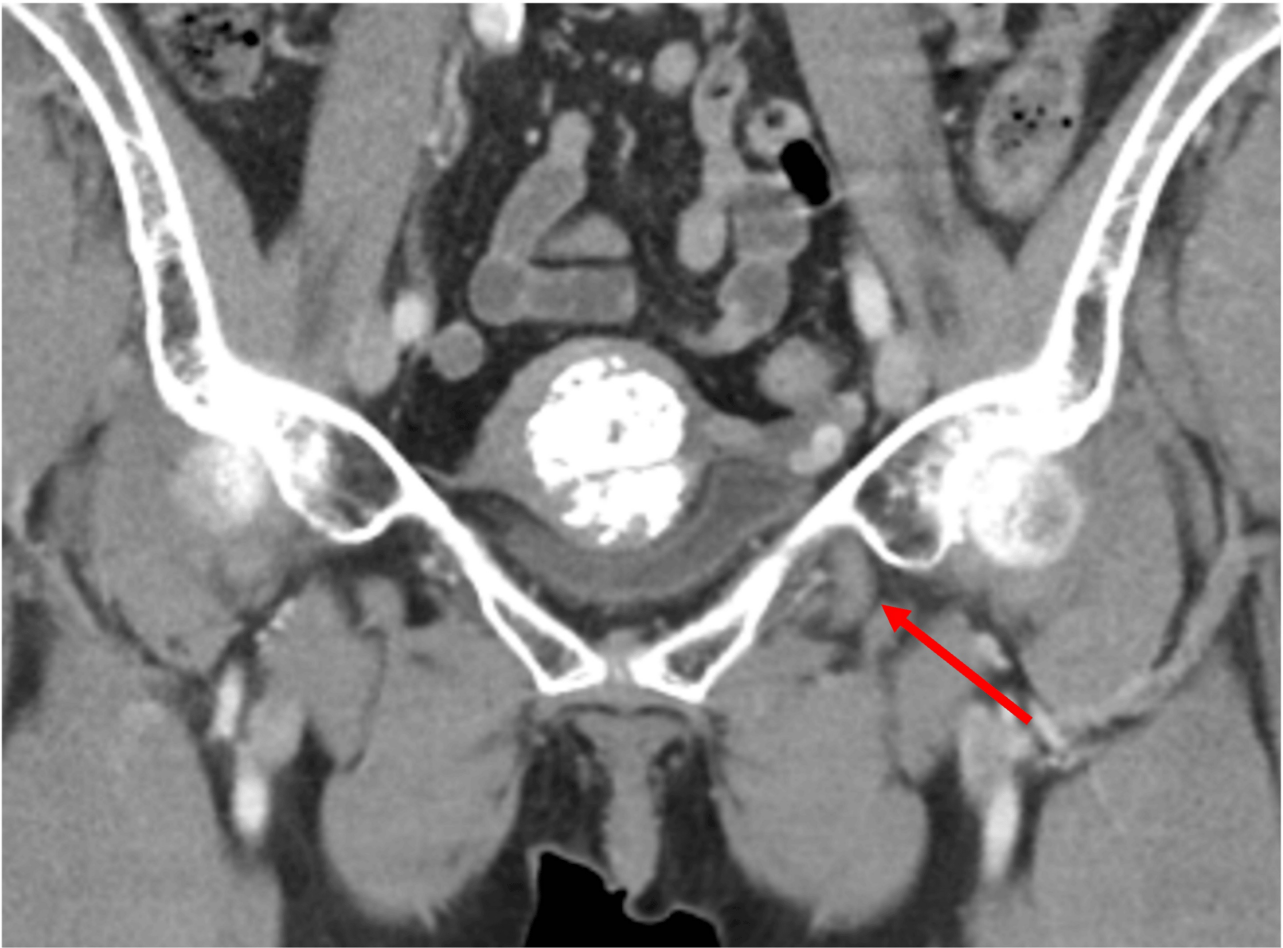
Contrast-enhanced coronal computed tomography image redemonstrating the decompressed diverticula as it passes through the obturator foramen.
